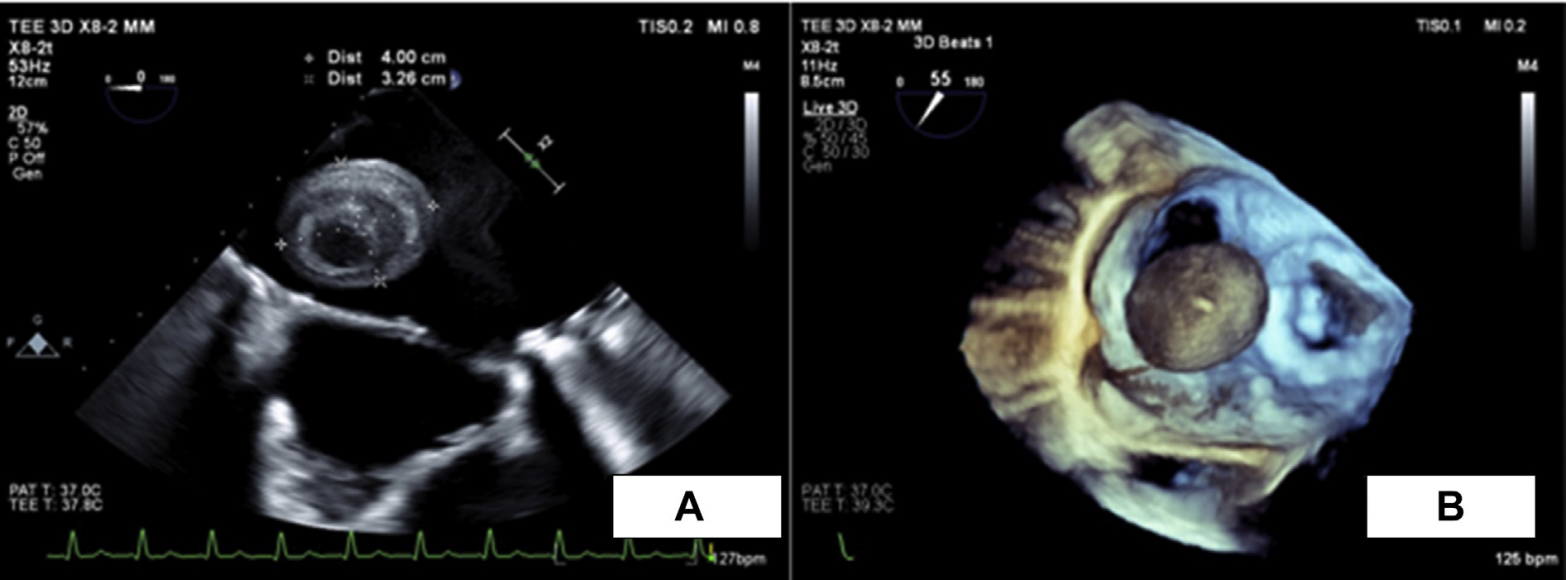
(A) Transesophageal echocardiography: two-dimensional midesophageal view showing giant LA thrombus. (B) Transesophageal echocardiography: three-dimensional zoomed midesophageal view demonstrating LA thrombus.
